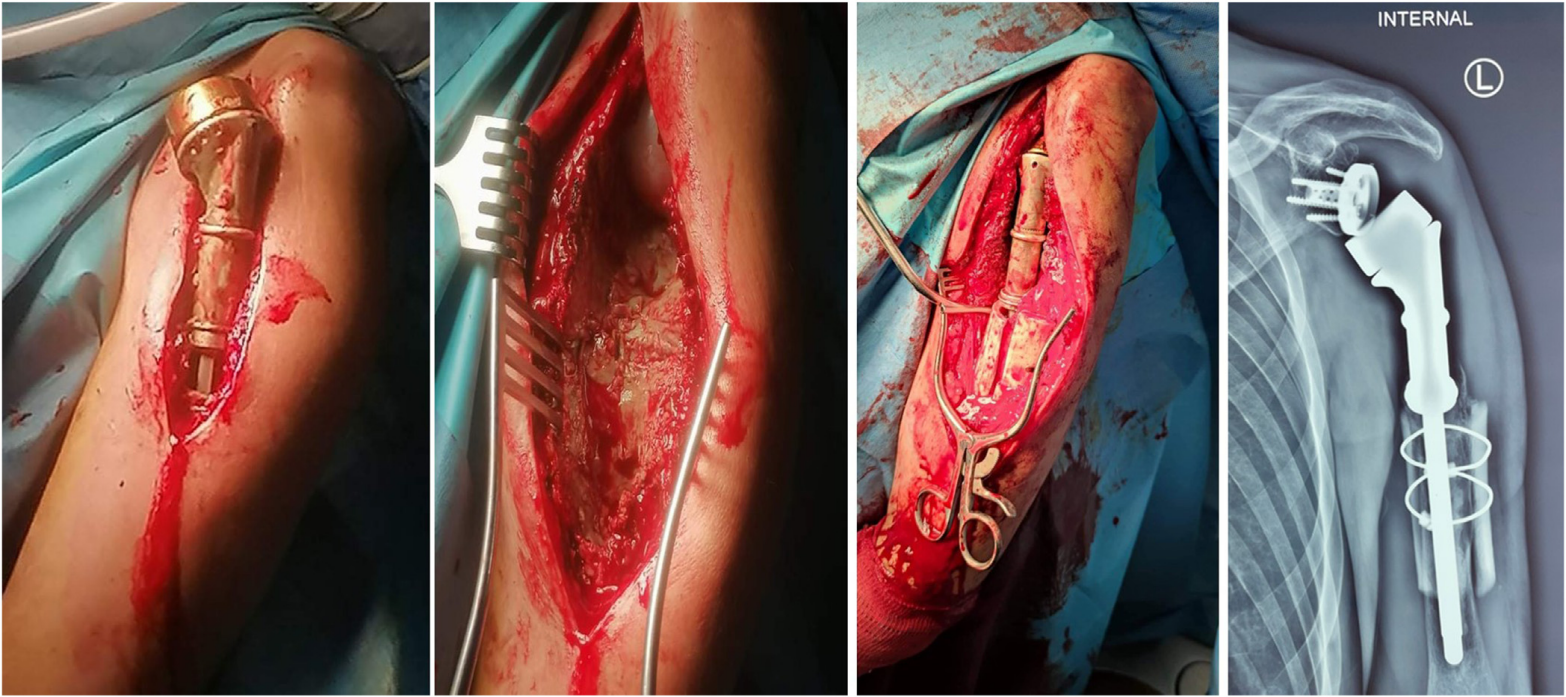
Intraoperative photos of the revision operation and postoperative X-ray showing the longer stem and the splitted allograft.
